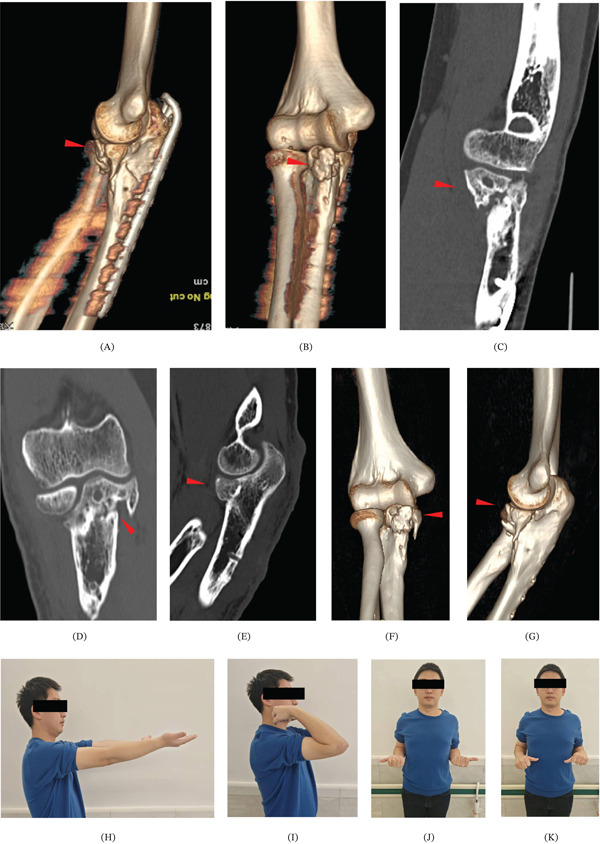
Postoperative follow‐up radiographic and clinical outcomes. (A–C) Three‐dimensional CT reconstructions and sagittal CT image obtained at 3‐month follow‐up, showing the coronoid process fracture site (red arrowheads) with early bone callus formation and the bone tunnel created by the all‐suture anchor. (D–G) Three‐dimensional CT reconstructions and coronal/sagittal CT images obtained at 1‐year follow‐up, after removal of the internal fixation plate, demonstrating complete osseous union of the coronoid process and olecranon fractures (red arrowheads) with restoration of normal humeroulnar joint congruity and no evidence of posttraumatic arthritis, nonunion, or implant‐related complications. (H–K) Clinical photographs at 1‐year follow‐up demonstrating full functional recovery of the elbow, including (H) full extension, (I) full flexion, and (J, K) symmetric forearm pronation and supination compared with the contralateral side.
